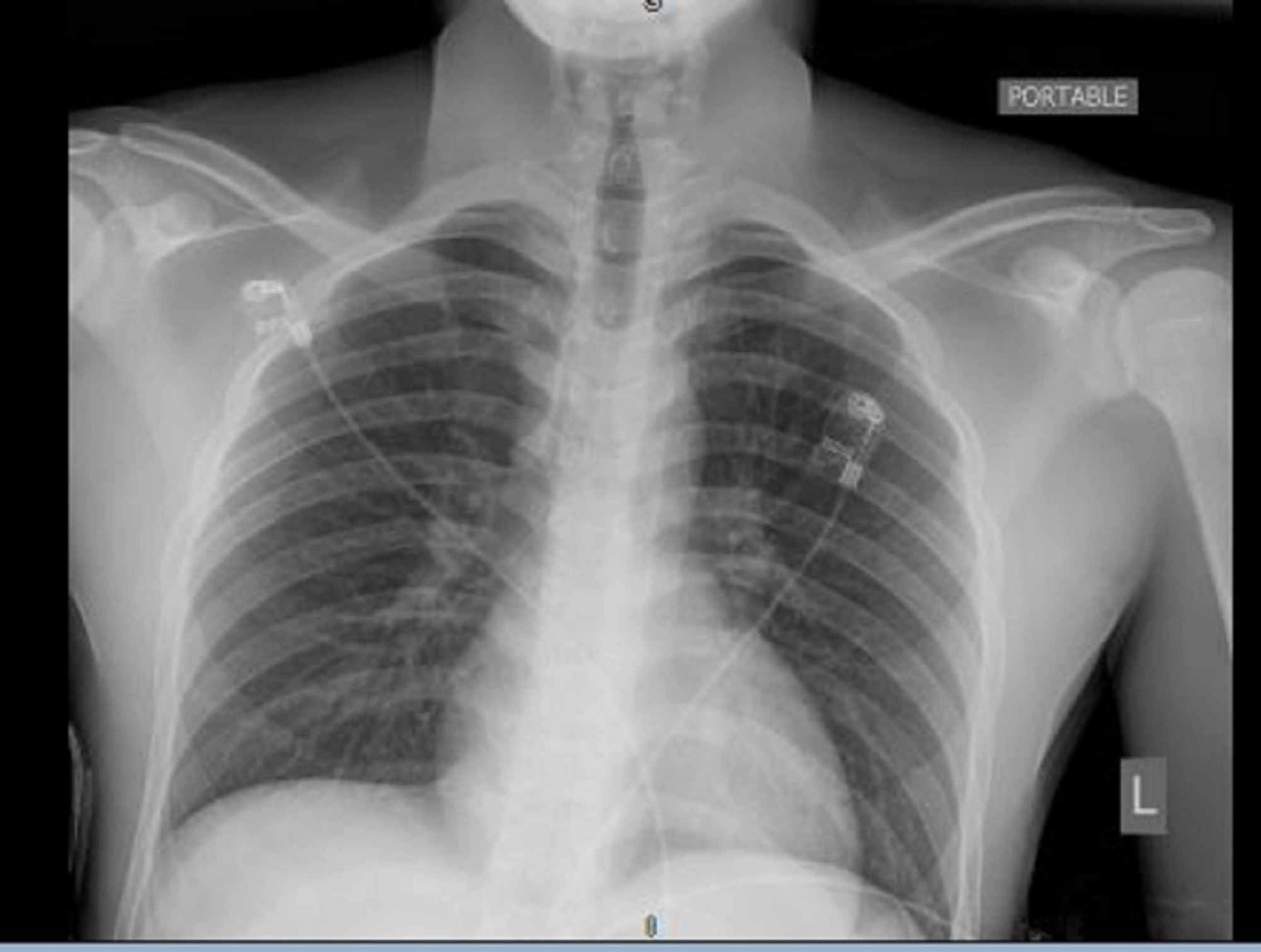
Portable chest X-ray on admission revealing no abnormal signs.
